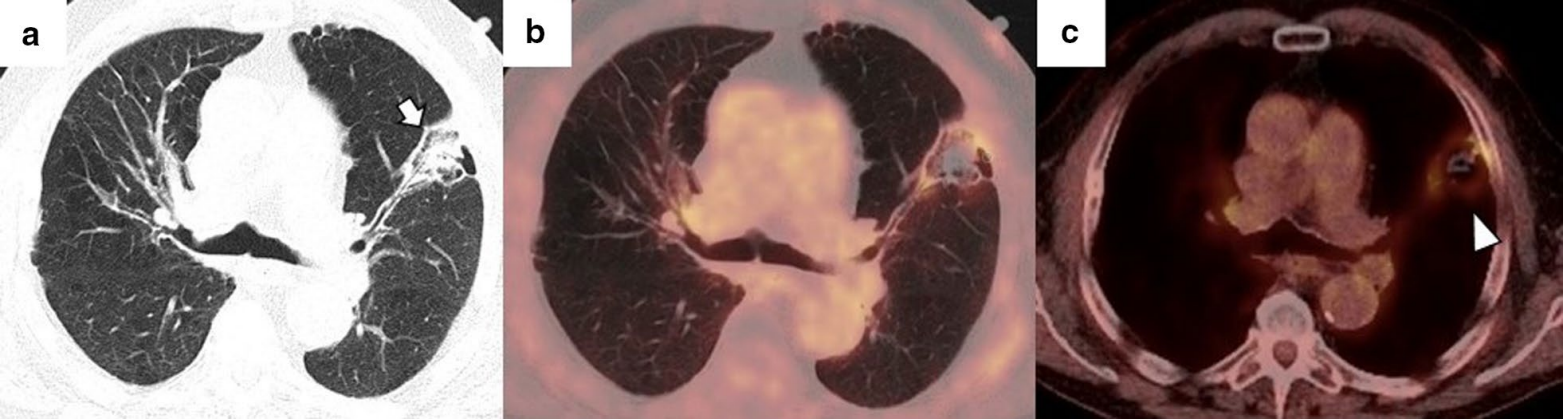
Peripheral FDG capture pattern on a PET/CT performed two months after image-guided percutaneous lung ablation. a non-contrast CT showing the treated area: a central consolidation surrounded by a peripheral ground-glass opacity with a tendency to consolidate (arrow). b, c PET/CT demonstrating peripheral FDG uptake of the treated area, a pattern suggestive of response to treatment
